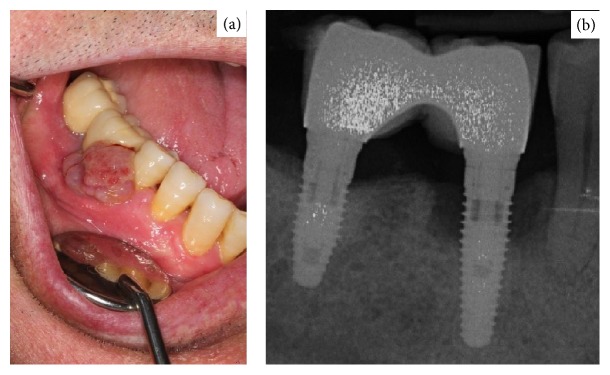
(a) Exophytic granulomatous lesion in 4.6. (b) Periapical radiograph that shows bone involvement in implant 46.
